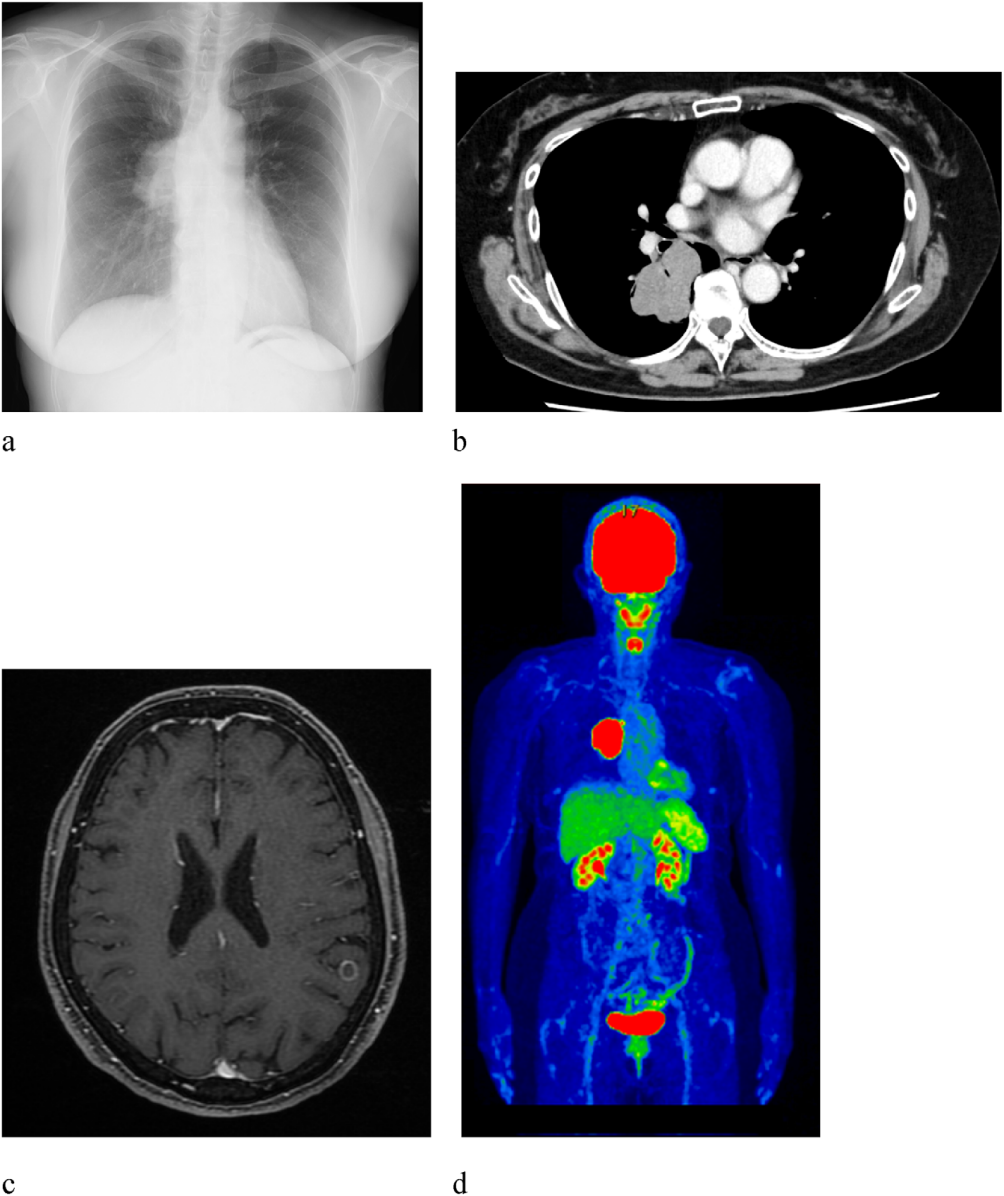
(a) Chest X‐ray, (b) contrast‐enhanced computed tomography (CT), (c) contrast‐enhanced head magnetic resonance imaging (MRI), and (d) positron emission tomography/computed tomography (PET/CT) at first admission. An irregular tumor was present in the right lower lobe, a metastatic brain tumor was detected in the left parietal lobe, and fluorodeoxyglucose uptake was noted. The maximum standardized uptake value (SUVmax) was 19.2
